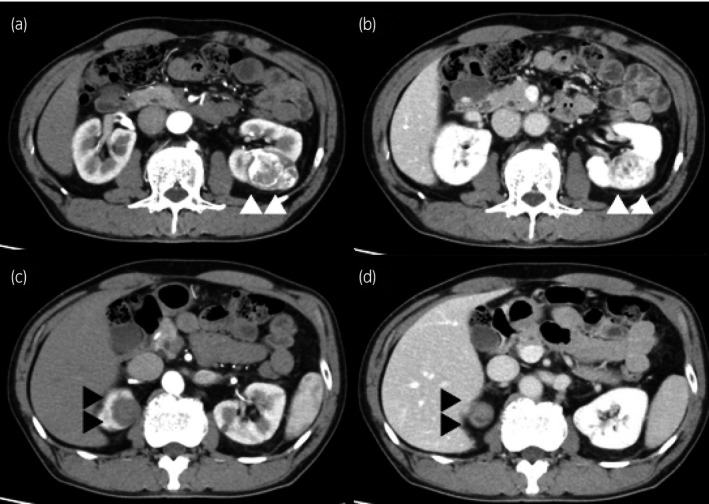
Preoperative CT of bilateral renal tumors. The left renal tumor is 42 mm in diameter and shows well‐defined margins and irregular contrast in the arterial phase (a) and early drainage in the renal parenchymal phase (b). The right renal tumor is 26 mm in diameter and shows well‐defined margins without contrast‐enhancement in both arterial and renal parenchymal phases (c,d).
